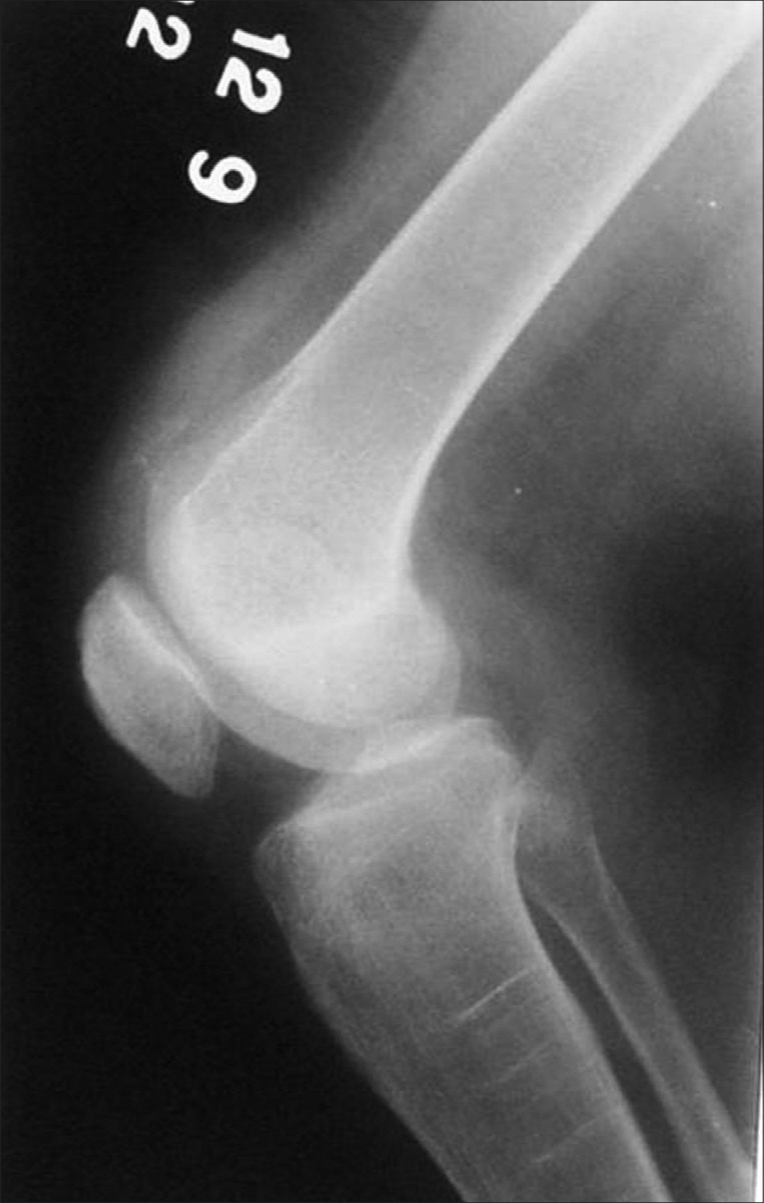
X-ray of right knee lateral view shows a slight inferior displacement of patella with loss of quadriceps tendon bulge just above it; calcification is seen in suprapatellar region
